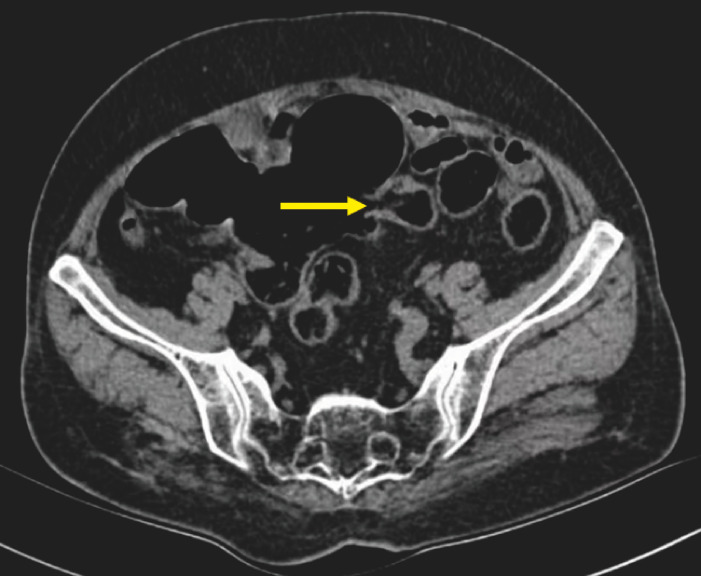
Computed tomography revealed a communication (arrow) between a portion of the small intestine and the adjacent segment of the sigmoid colon, with lumen dilatation and localized air and fluid accumulation.
